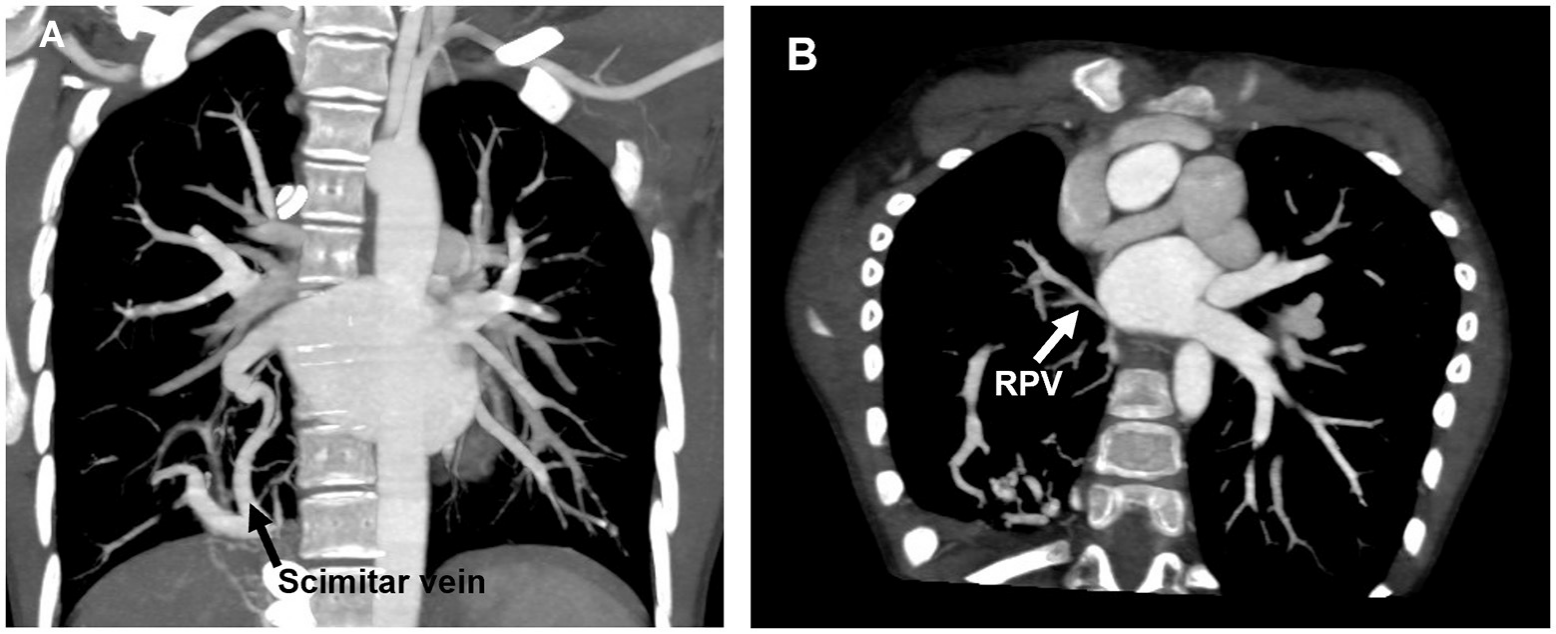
CT scan showing anomalous pulmonary venous drainage of the right lung to the IVC (A) and restenosis of RPV after operation (B). CT, Computed tomography; IVC, Inferior vena cava; RPV, Right pulmonary vein.
